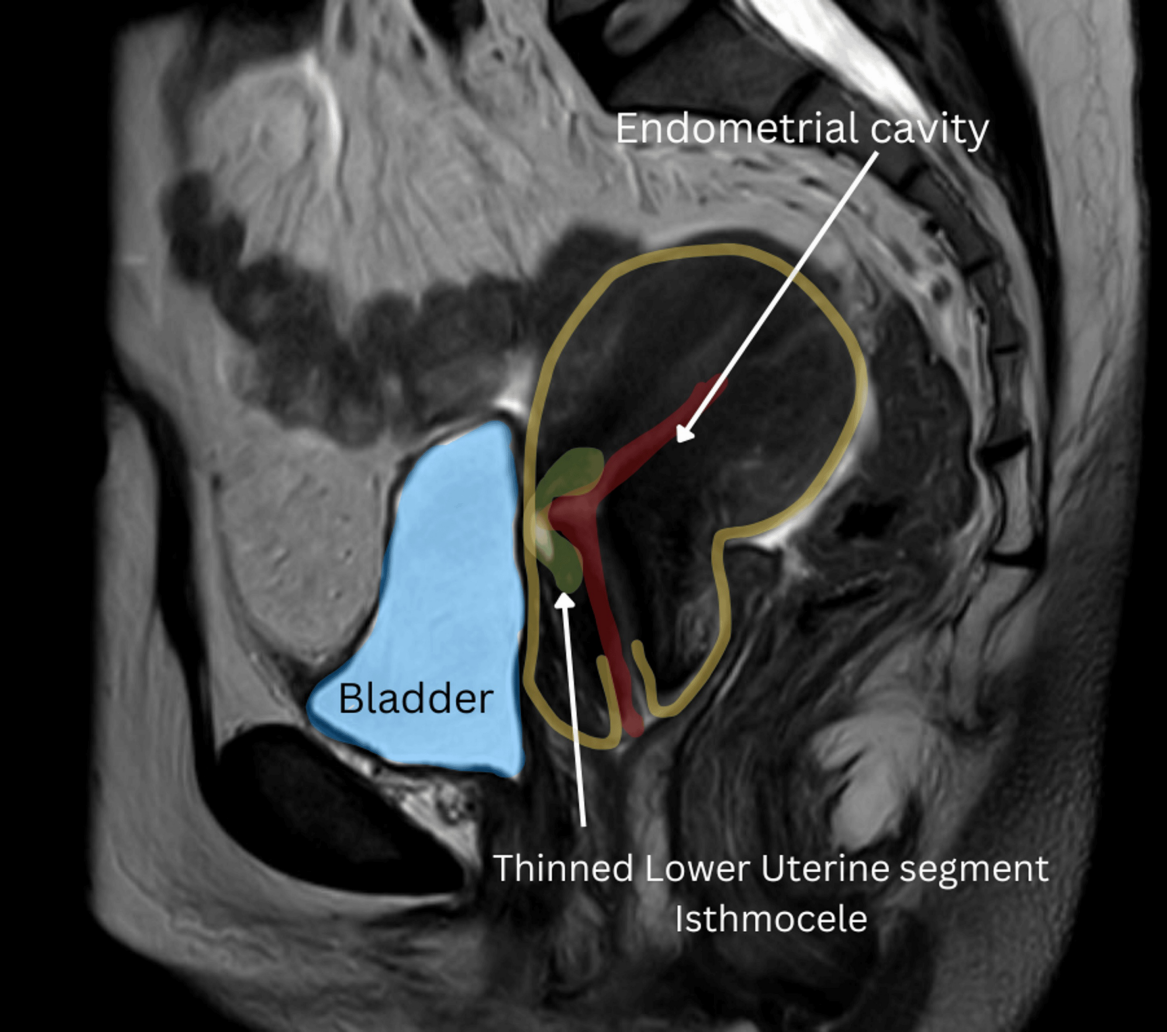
MRI visualization (T2-weighted sagittal section) of uterine isthmocele. The myometrial scar thickness is 3 mm. MRI: Magnetic resonance imaging
